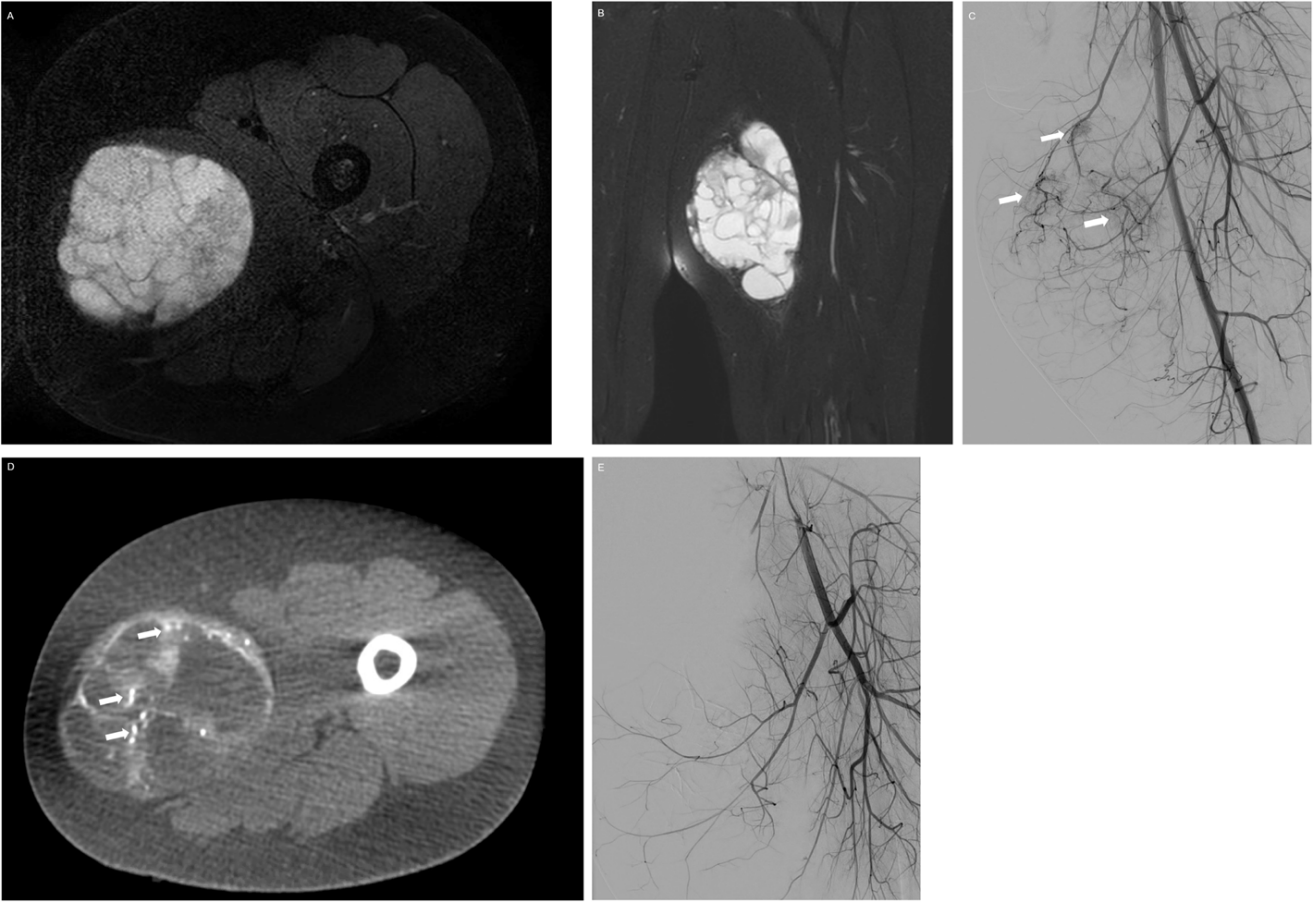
T2- weighted fat saturated axial (a) and coronal (b) MR image demonstrating a soft tissue sarcoma (malignant hemangiopericytoma) in the thigh of a 10-year-old male. A pre-resection embolization was requested due to its hypervascularity and risk of bleeding during surgery. c Pre-operative angiography demonstrating arterial tumor blush from branches of the superficial and deep femoral arteries (arrows). These feeders were embolized with 70–150 DC bead LUMI to reduce bleeding during resection. d Axial cone beam CT image obtained post embolization demonstrating the radio-opaque embolization beads in the tumor bed (arrows). e Post embolization angiography demonstrating disappearance of the arterial tumor blush
